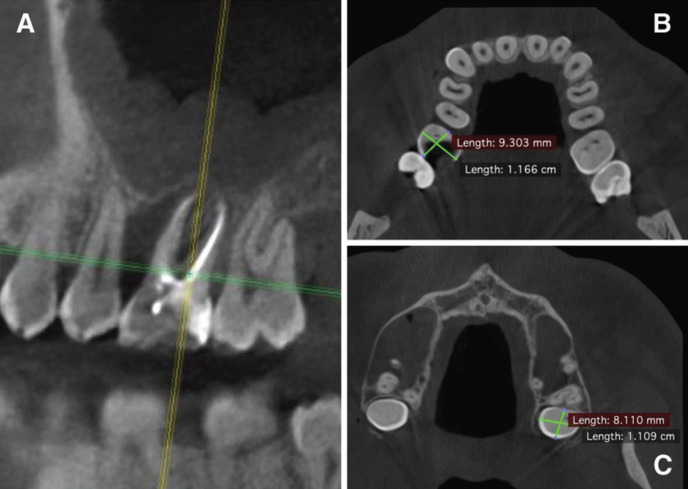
A. CBCT scans before surgery, showing vertical bone loss and inflammation in the maxillary sinus. B. Measurements of the extracted tooth. C. Measurements of the donor tooth.
